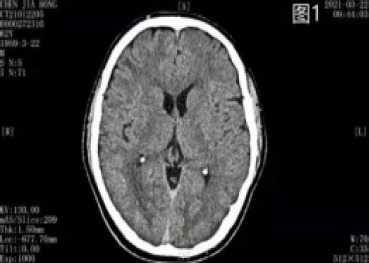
CT showed no obvious abnormality
